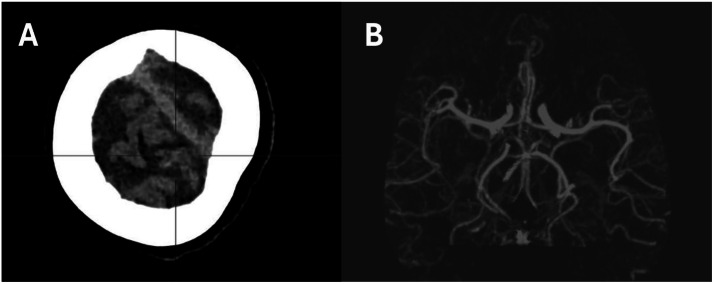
Images of the brain post-biopsy (A) CT scan showing pneumocephalus, (B) CT intracranial angiogram.
